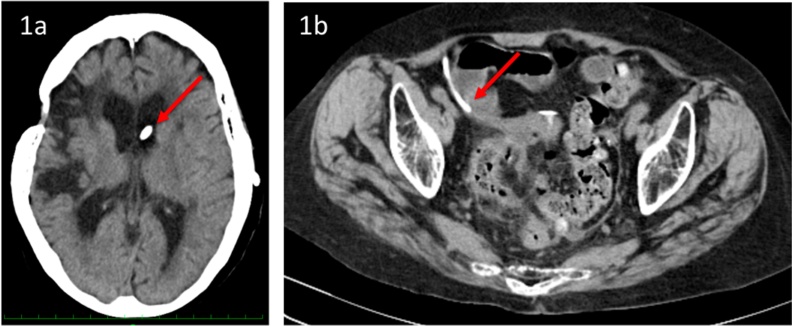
a) Head computed tomography (CT) revealed a low-density area in the right hemisphere indicating impairment due to aneurysmal subarachnoid hemorrhage, and the ventricular part of the ventriculoperitoneal (VP) shunt was inserted into the left ventricle (Red arrow). B) Abdominal CT revealed a diverticular sigmoid colon surrounded by free air and isodensity ascites, and the peritoneal part of the VP shunt was placed far from the sigmoid colon (Red arrow).
